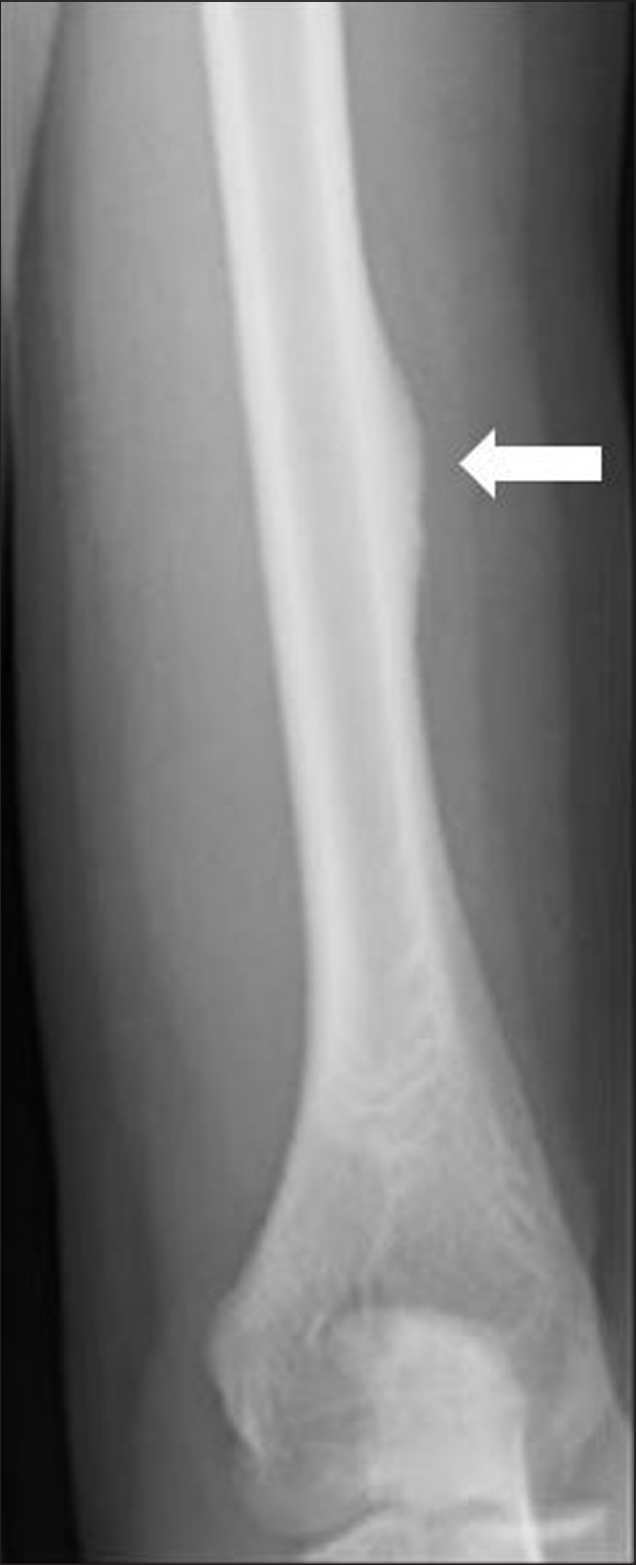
Magnetic resonance imaging (MRI) revealed a linear area of high signal intensity at the periosteal side of the humeral shaft as well as adjacent muscle oedema at the insertion of the brachialis muscle on Fat suppressed (FS) T2-weighted image (WI) (*Figure 2*, arrow).
